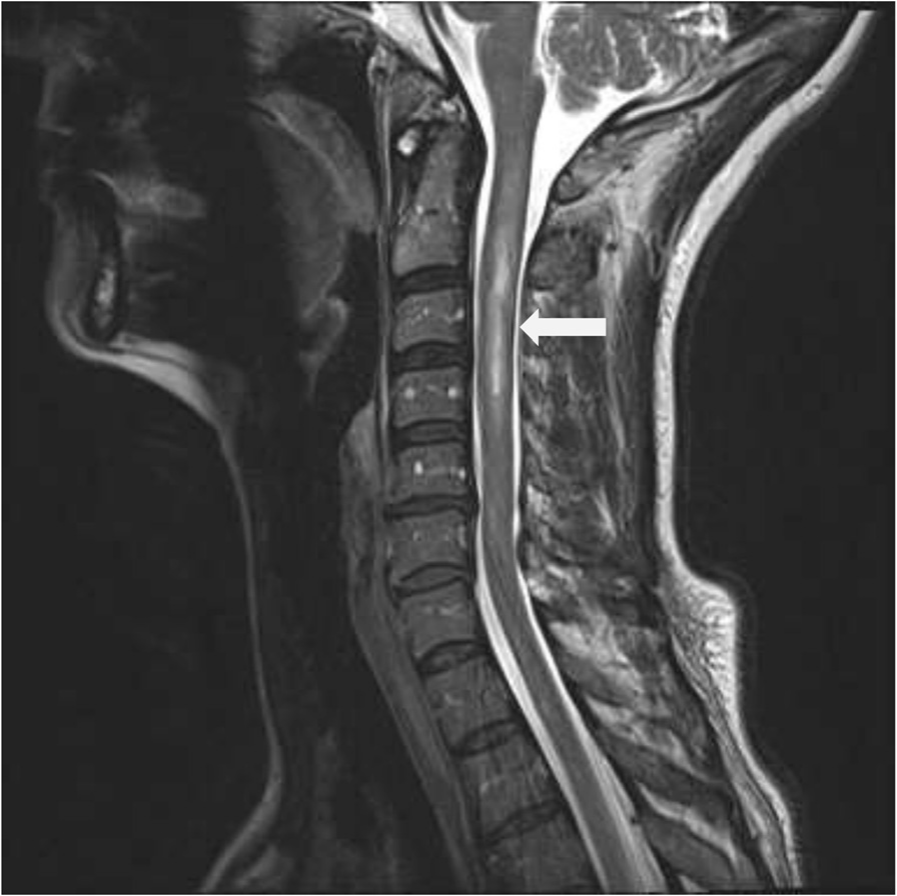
MRI T2 weighted sequence of the cervical region showing contrast enhancing lesion from C2-C4.
